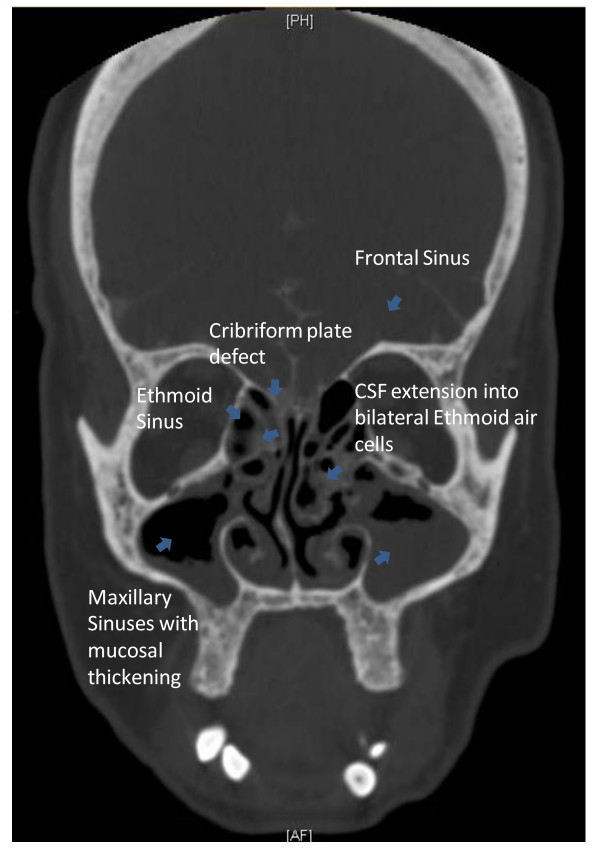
Preoperative CT scan of the head. A preoperative CT scan of the head with intrathecal contrast was done to localize the CSF leak. The frontal view is shown here. The scan shows a defect in the cribriform plate with confluent CSF extension into several bilateral ethmoid air cells, evidenced by the higher intensity signal of the contrast. Secondarily, there is mucosal thickening in the ethmoid and maxillary sinuses.
